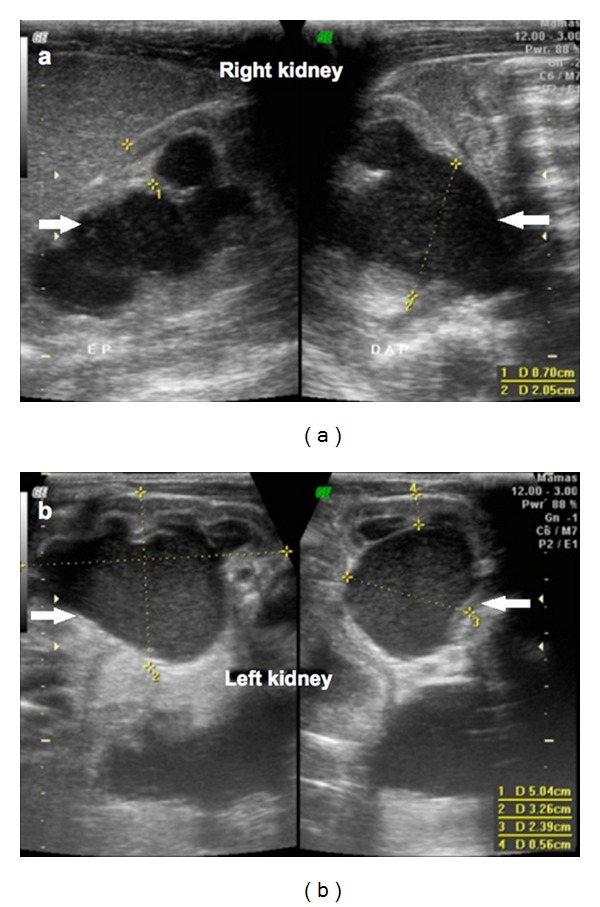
Ultrasound scan in sagittal plane at the level of the kidneys shows bilateral hydronephrosis of dense content (white arrows): (a) in the right kidney; (b) left kidney.
